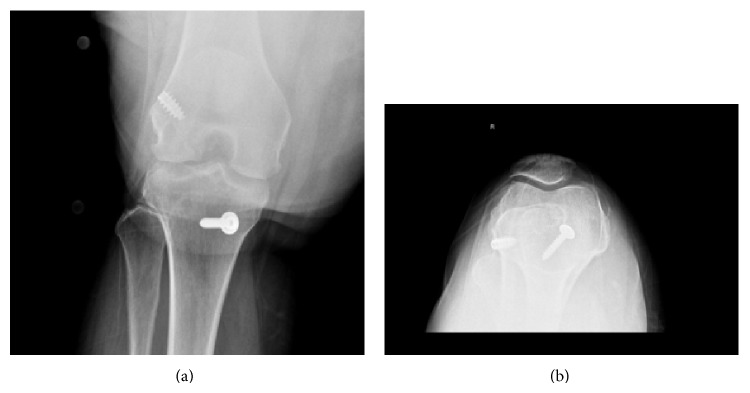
(a) Anteroposterior (AP) and (b) sunrise view of the right knee in a 39-year-old female patient who underwent ACL reconstruction with medial meniscectomy at the age of 33 after sustaining an ACL tear with concomitant medial meniscus and MCL tears. These radiographs demonstrate joint space narrowing, particularly in the medial and patellofemoral compartments, consistent with early osteoarthritic changes.
